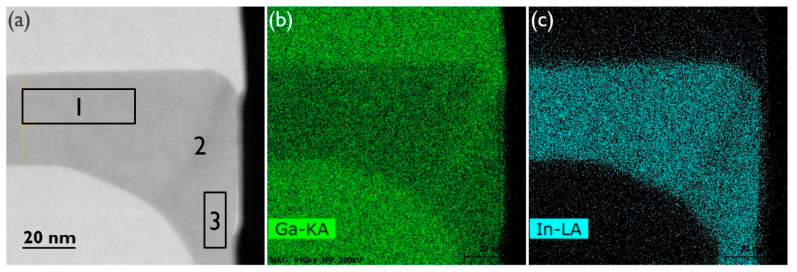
(a) HAADF-STEM image of an InGaP corner between 2 GaAs layers, and EDS maps of that corner based on (b) Ga-Kα and (c) In-Lα X-ray emission. The In composition of regions 1, 2 (the darker vein) and 3 is deduced as the following: 42%, 33% and 40%, respectively.
